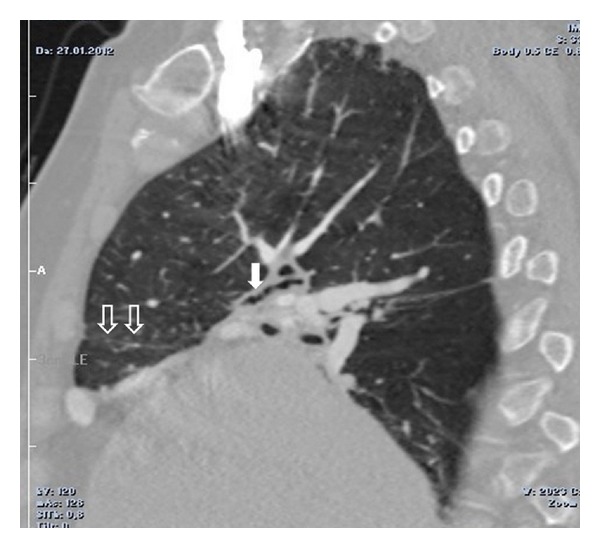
On sagittal CT image, hypoplasia of the right intermediate and middle lobe bronchus (white arrow), parenchymal scars and loss of ventilation in the middle lobe of the right lung were seen (open arrows).
